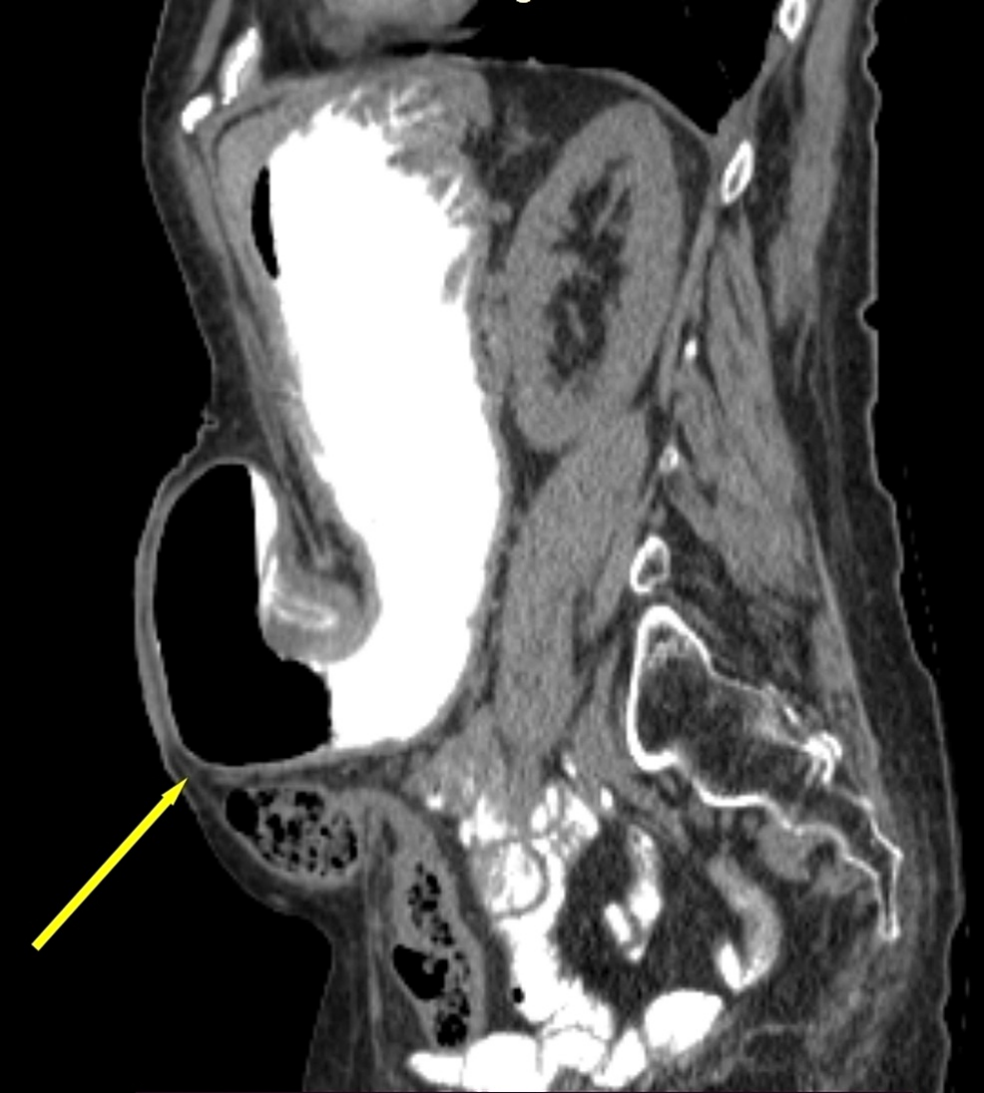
Sagittal view in abdominal CT scan, yellow arrow indicates stomach herniation.
